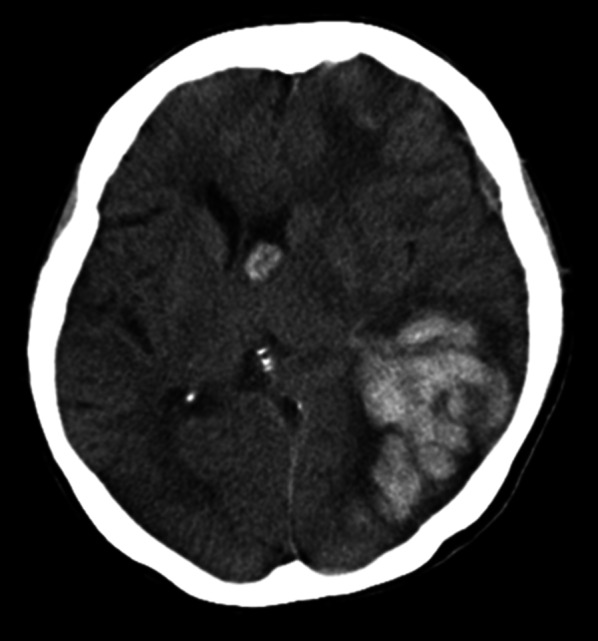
CT scan on the day when the patient developed consciousness disorder. Bleeding near the posterior horn of the left lateral ventricle was prominently enlarged to 8 cm in diameter, and left uncus herniation and subfalcian herniation were evident
